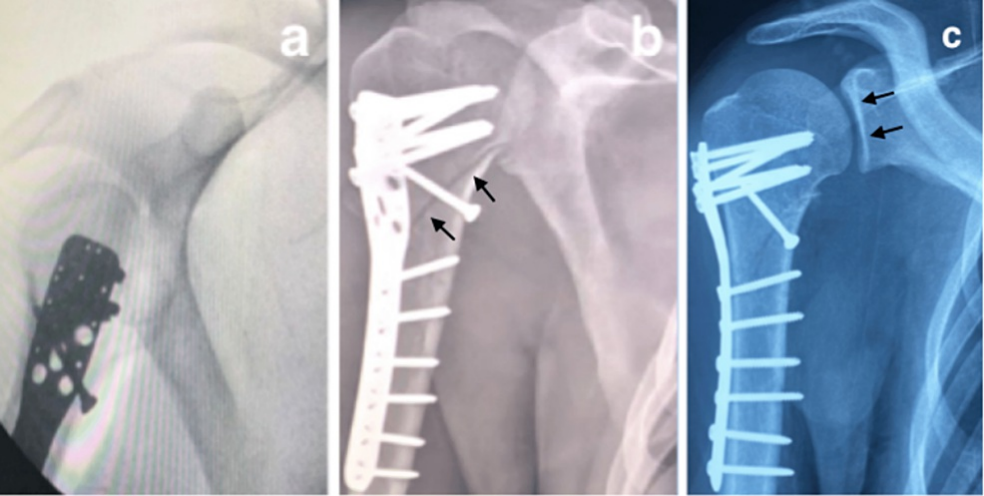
Intraoperative (a), postoperative (b), and three-week radiographs showing good reduction of the posterior shoulder fracture-dislocation (black arrow).
